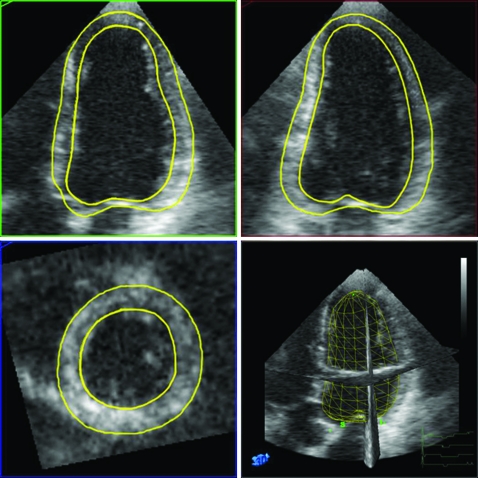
End-diastolic, anatomically correct apical four-chamber and two-chamber views (upper left and right, respectively) and short-axis view (bottom left) selected off-line from a 3-DE dataset obtained in the same subject and resulting 3D-shell (bottom right). Superimposed semi-automatically detected endocardial and epicardial boundaries were used to calculate LV mass.
